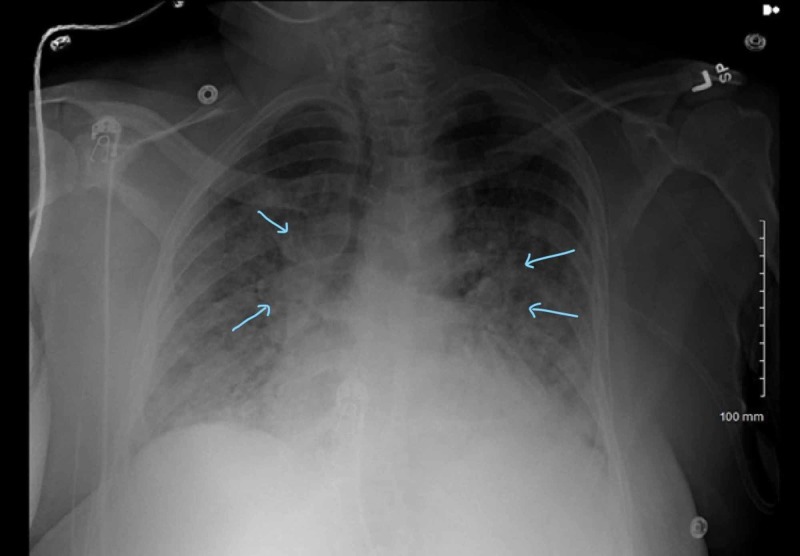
Chest X-ray suggestive of bilateral pulmonary edema, more on the right compared to left (arrows pointing)
